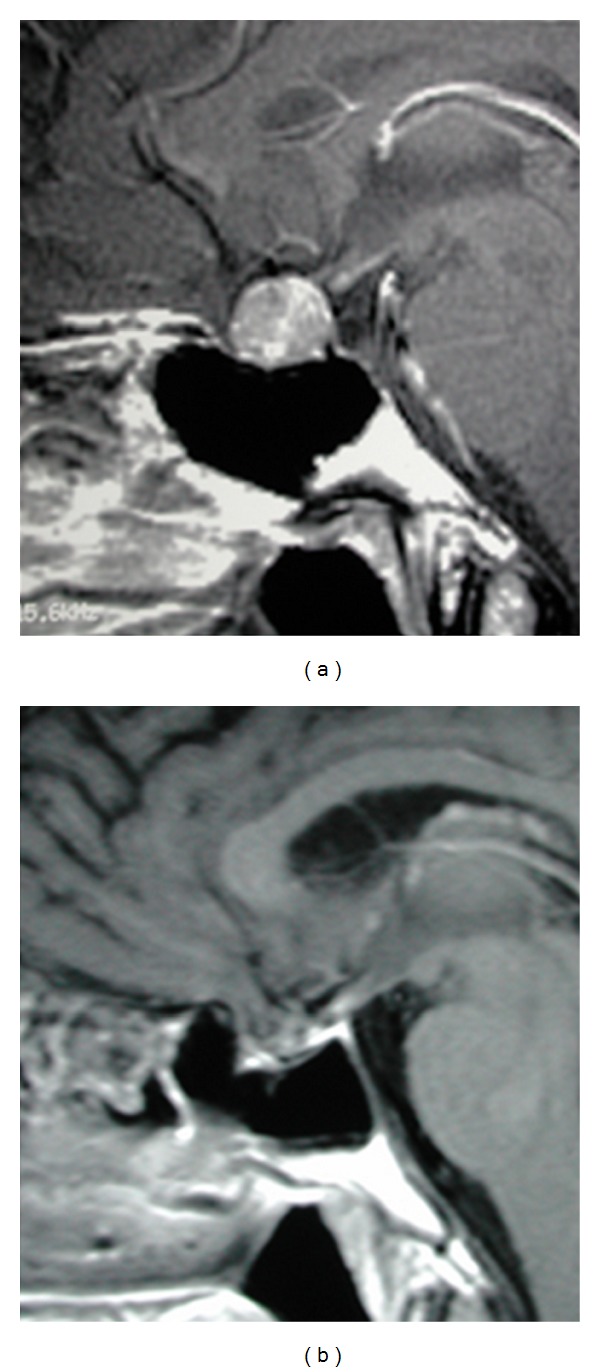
Contrast enhanced MRI sagittal view showing an intrasellar pituitary adenoma (a) that could be completely removed with surgery (b). Biochemical remission was also gotten.
